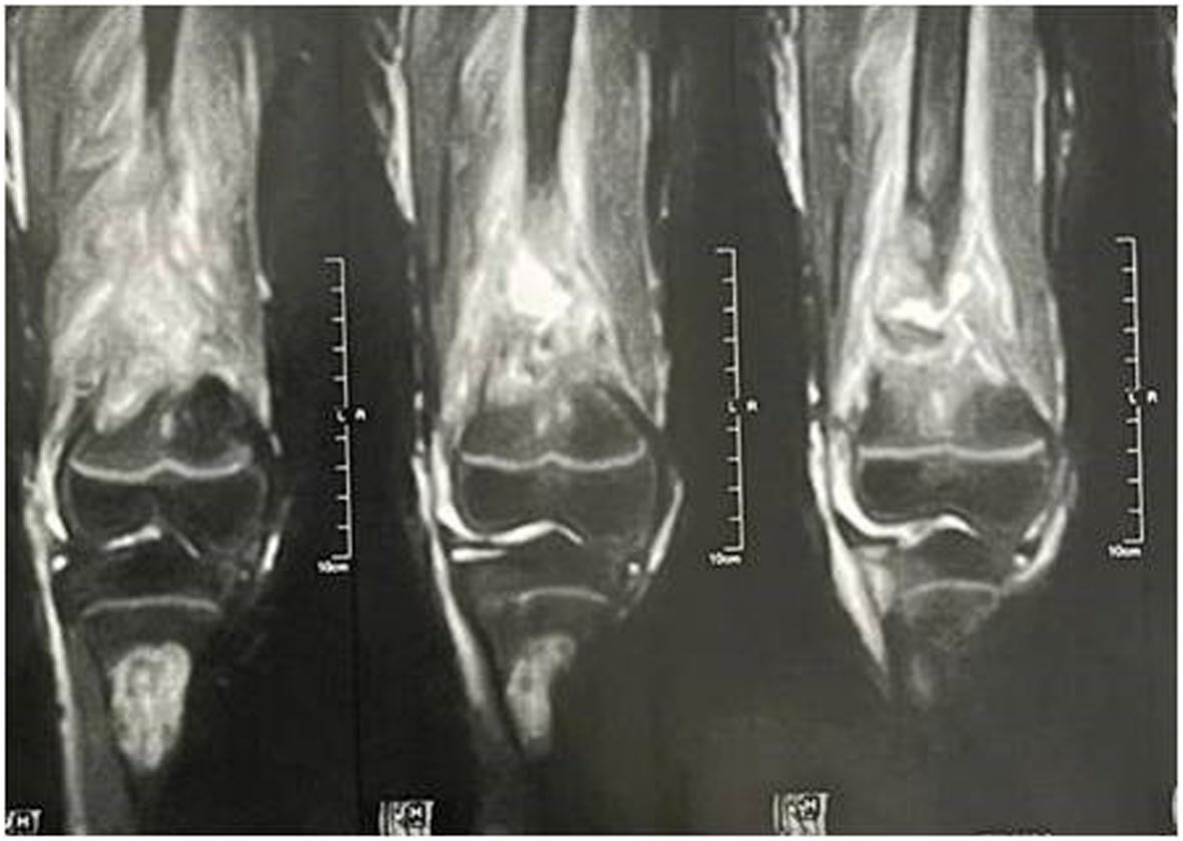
MRI of the NOF in the distal femur and proximal tibia. The Distal femur is surrounded by effusion due to the fracture
